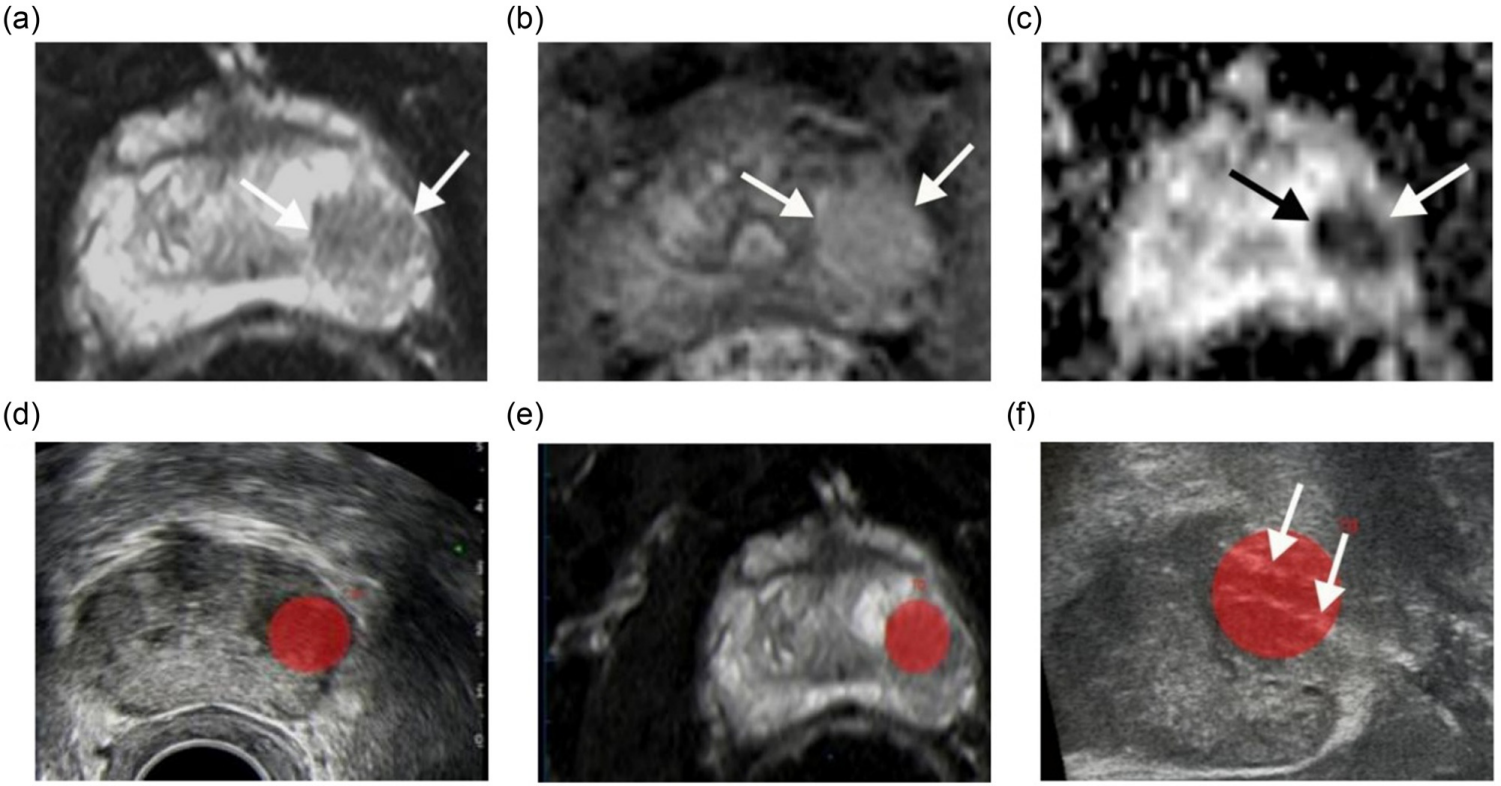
Fusion imaging and biopsy. A lesion was shown on mpMRI. Example of a 74 year old male (PSA 17.1 ng/mL) with a PI-RADS 5 lesion at 4 o’clock (white arrows) covered PZ and TZ: (a)–(c) T2, DCE, and ADC of a solid (arrowhead) are seen; (d) and (e) DWI image was uploaded on the ultrasound machine and coupling for MRI–TRUS fusion biopsy; the lesion was not obvious on the ultrasound images; (f) two biopsy needle marks were seen in target area (arrowhead).
